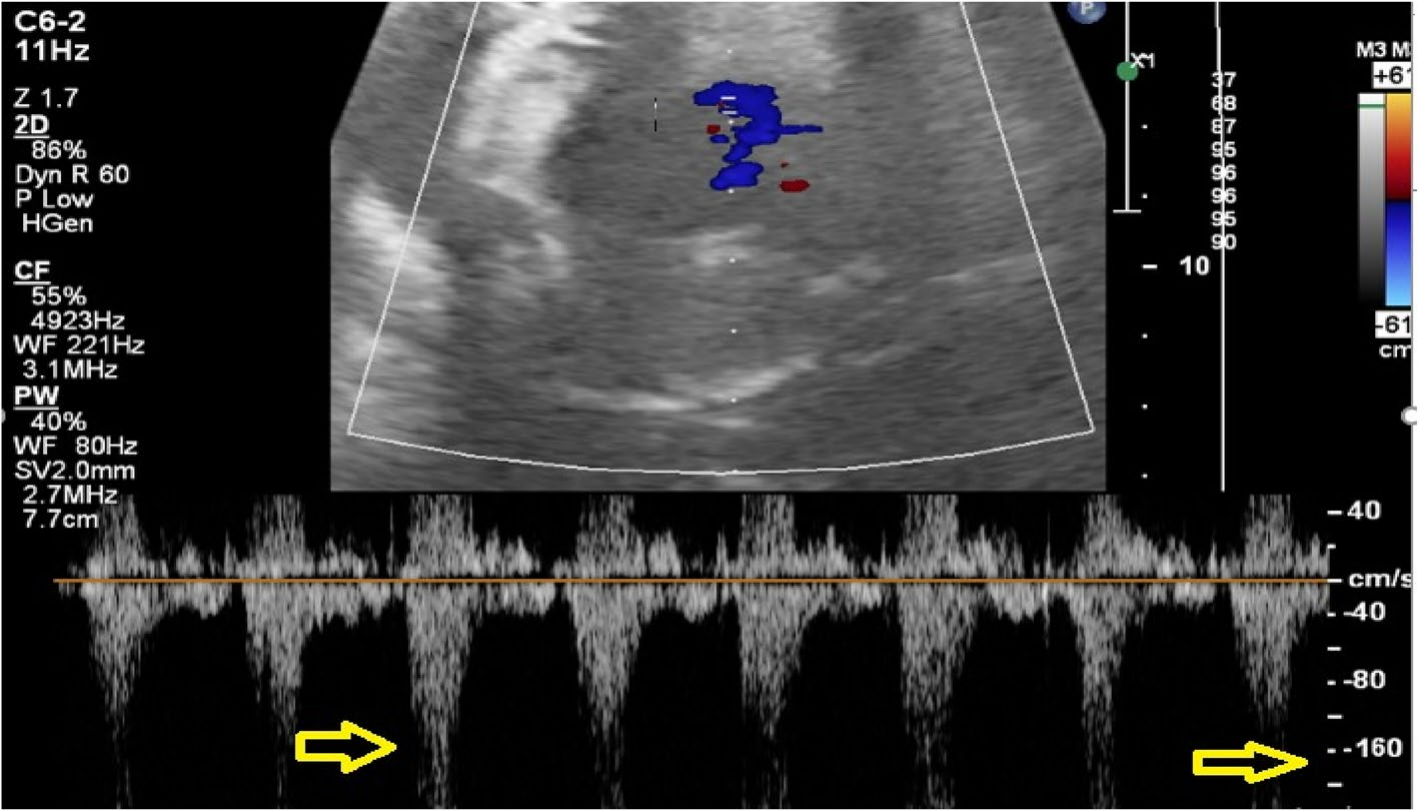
Doppler spectral in pulmonary artery shows severe pulmonary valve stenosis
